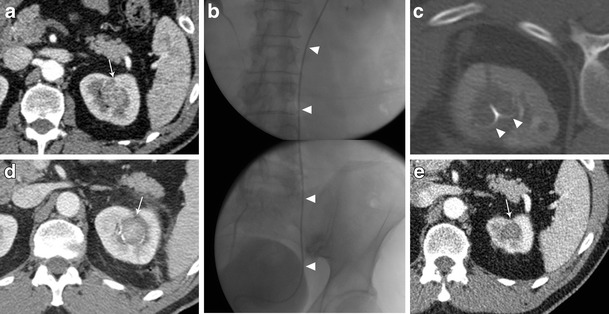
Treatment of a left kidney central tumour with renal sinus extension. a contrast enhanced CT demonstrating a centrally located tumour (*white arrow*) with extension in close proximity of the renal sinus. b retrograde pyeloperfusion was performed through a single j stent (*arrowheads*) placed endoscopically the day of the ablation. c CT scan after insertion on a radiofrequency electrode (*arrowheads*) into the tumour. d contrast enhanced CT performed immediately after the ablation demonstrated the complete ablation of the tumour (*white arrowhead*) without complications. e Contrast enhanced CT 2 years after the ablation demonstrating complete ablation with tumour shrinkage and no complications
